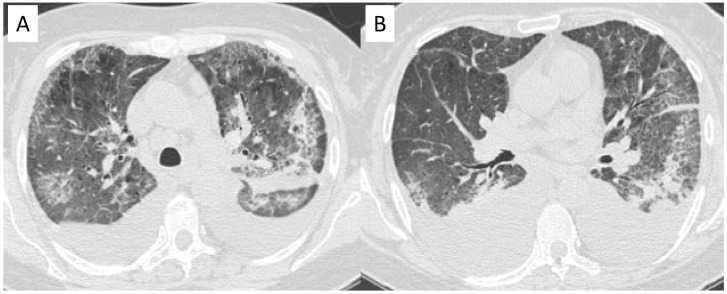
Cryoglobulinemic vasculitis in a patient with chronic renal failure and dyspnoea. Axial HRCT images show diffuse areas of ground-glass attenuation, due to alveolar hemorrhage, with interlobular and intralobular septal thickening. Pleural effusions are also shown in the figures (A,B).
